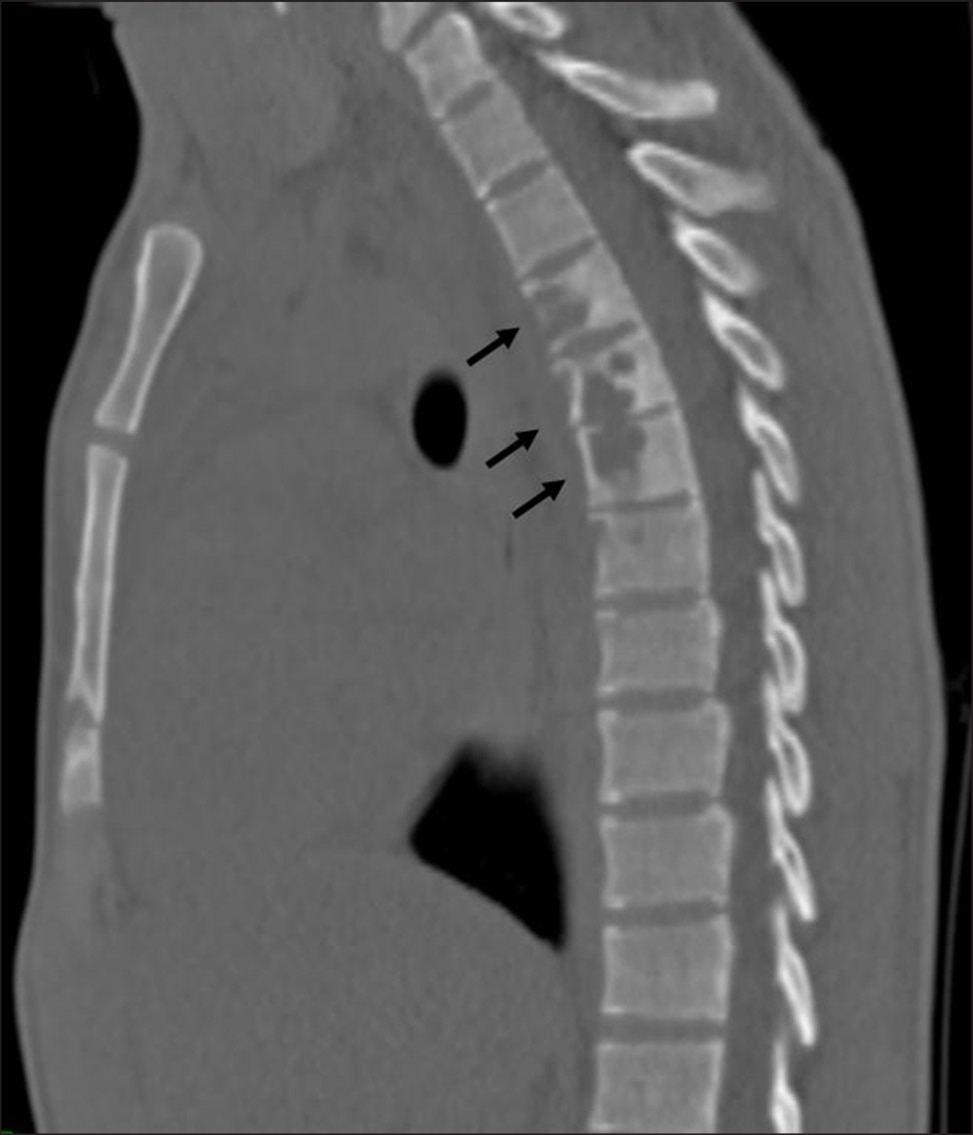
Reformatted sagittal CT scan image of the chest (bone window), showing extensive erosion involving the anterior aspect of three mid thoracic vertebral bodies (arrows). Note the subtle interrupted sclerotic margins as well as slight loss of height of the intervertebral disks in the affected segments.
